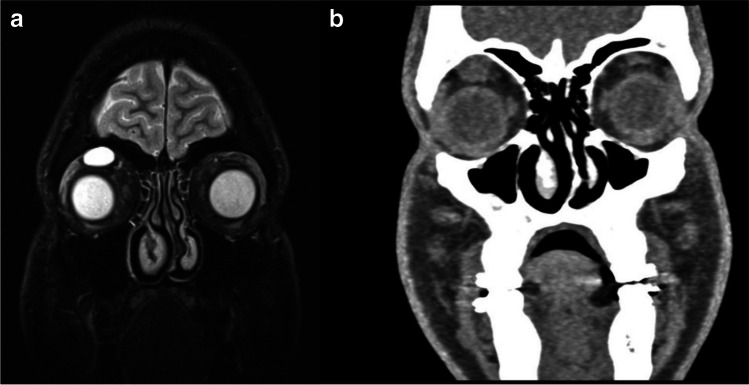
a) MRI on presentation supporting the diagnosis of orbital schwannoma (Patient 5), b) CT after 5 years showing no growth of the mass during the observation period
